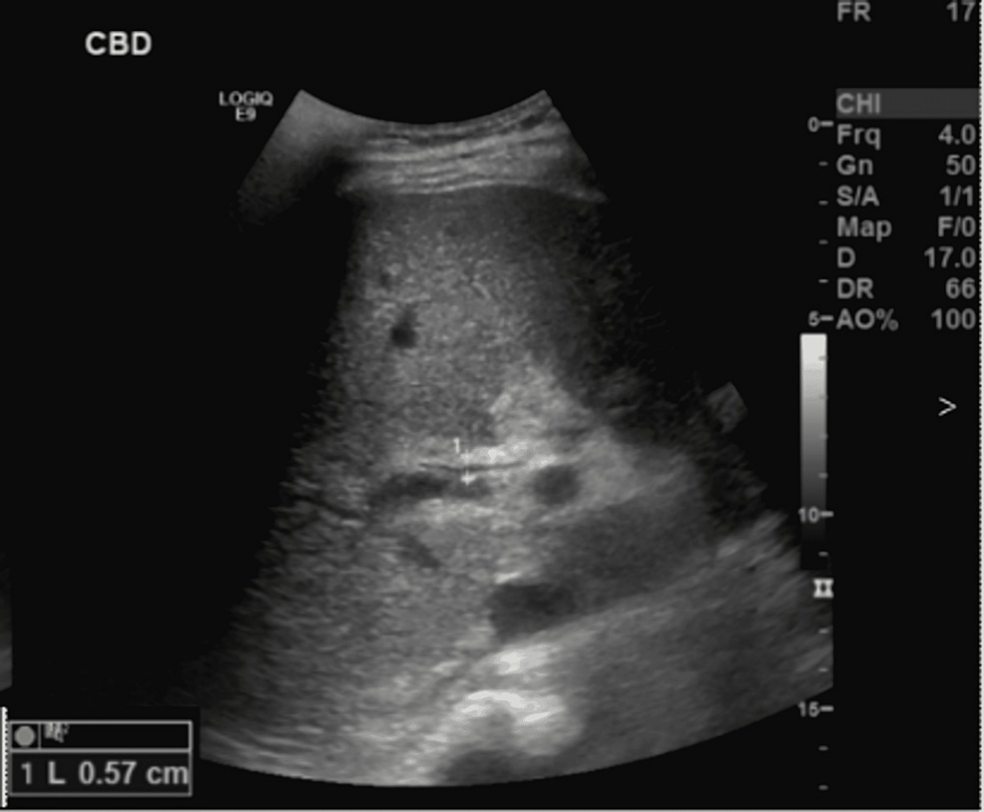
Post-ERCP ultrasound of the abdomen: CBD 5.7 mm
